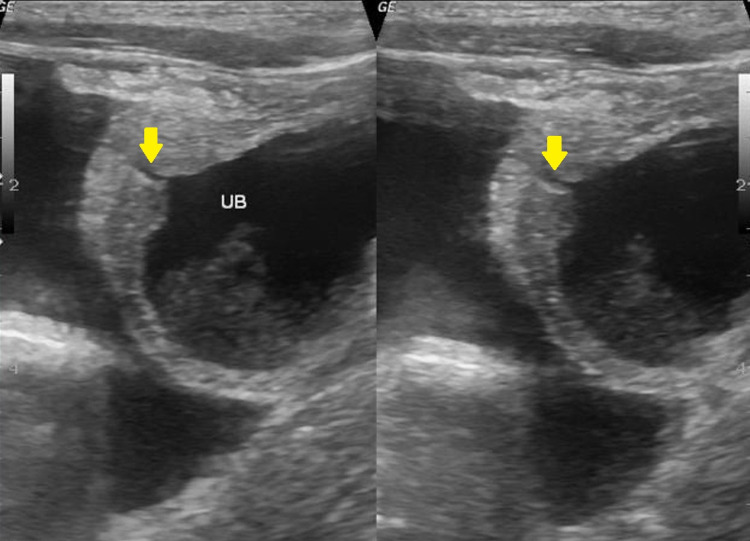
High-resolution ultrasonography image of the pelvis demonstrating intraperitoneal free fluid with evidence of hypoechoic disruption (arrow) involving the dome of the urinary bladder. UB: urinary bladder.
